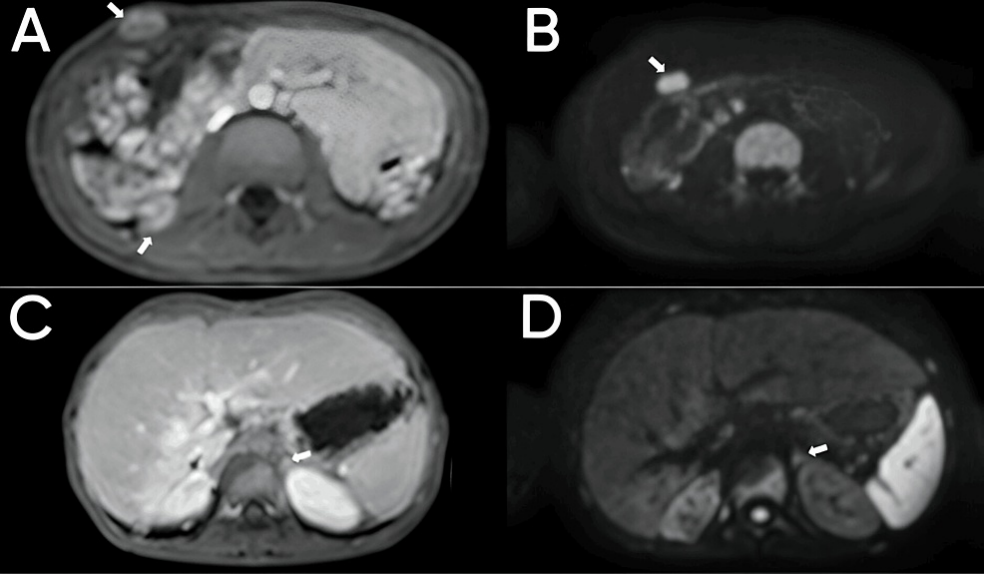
Abdominal magnetic resonance imaging with intravenous contrast in the axial plane following contrast administration (A and C) and diffusion-weighted sequence with B 800 (B and D). Nodular lesions in the abdominal wall and the right psoas muscle enhancing with contrast medium (arrows in A) and restricting diffusion (arrows in B) consistent with tumoral implants. The left adrenal gland appears normal in size and enhancement with contrast medium (arrow in C), without diffusion restriction foci (arrow in D).
